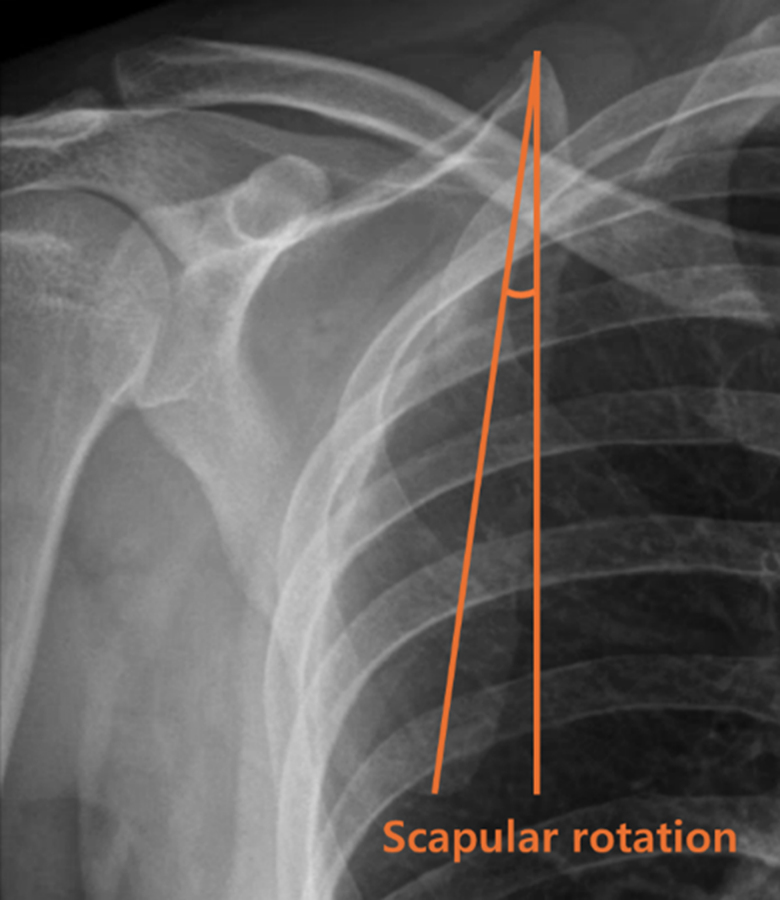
Measurement of scapular rotation angle on an anteroposterior chest radiograph. Scapular rotation angle was determined by measuring the angle formed between a vertical reference line and a line connecting the superior and inferior angles of the scapula.
